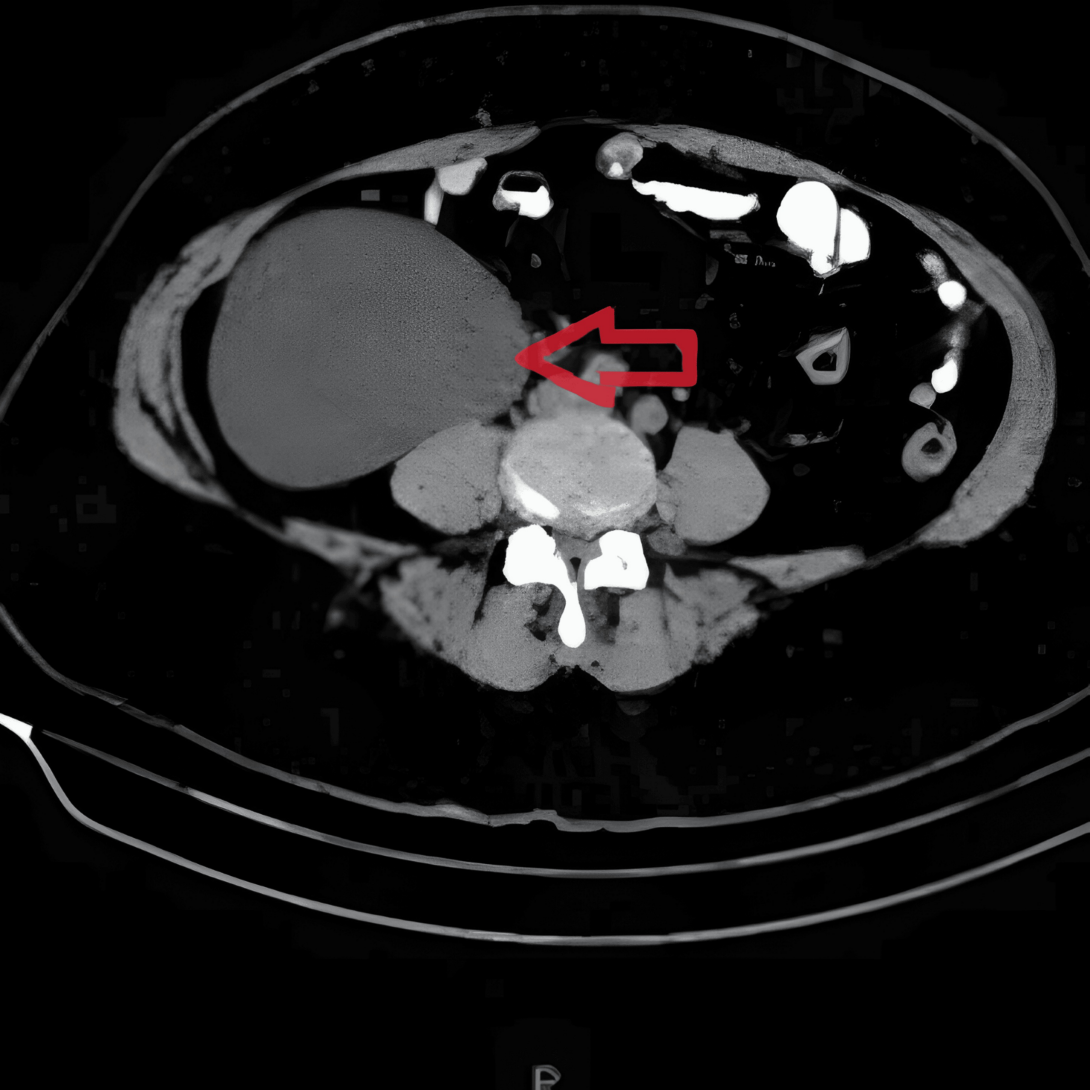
Axial view of CECT showing a right mesenteric cyst (arrow) CECT, contrast-enhanced CT
